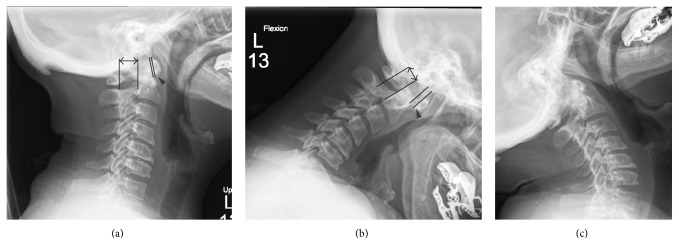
Lateral radiographs of a patient with atlantoaxial instability. In the neutral view the AADI (*arrowhead)* is 1 mm and the PADI (*double arrow)* is 20 mm (a). In flexion the AADI increases to 7 mm and the PADI decreases to 13 mm (b). In extension the AADI and PADI reduce to their neutral measures (c).
